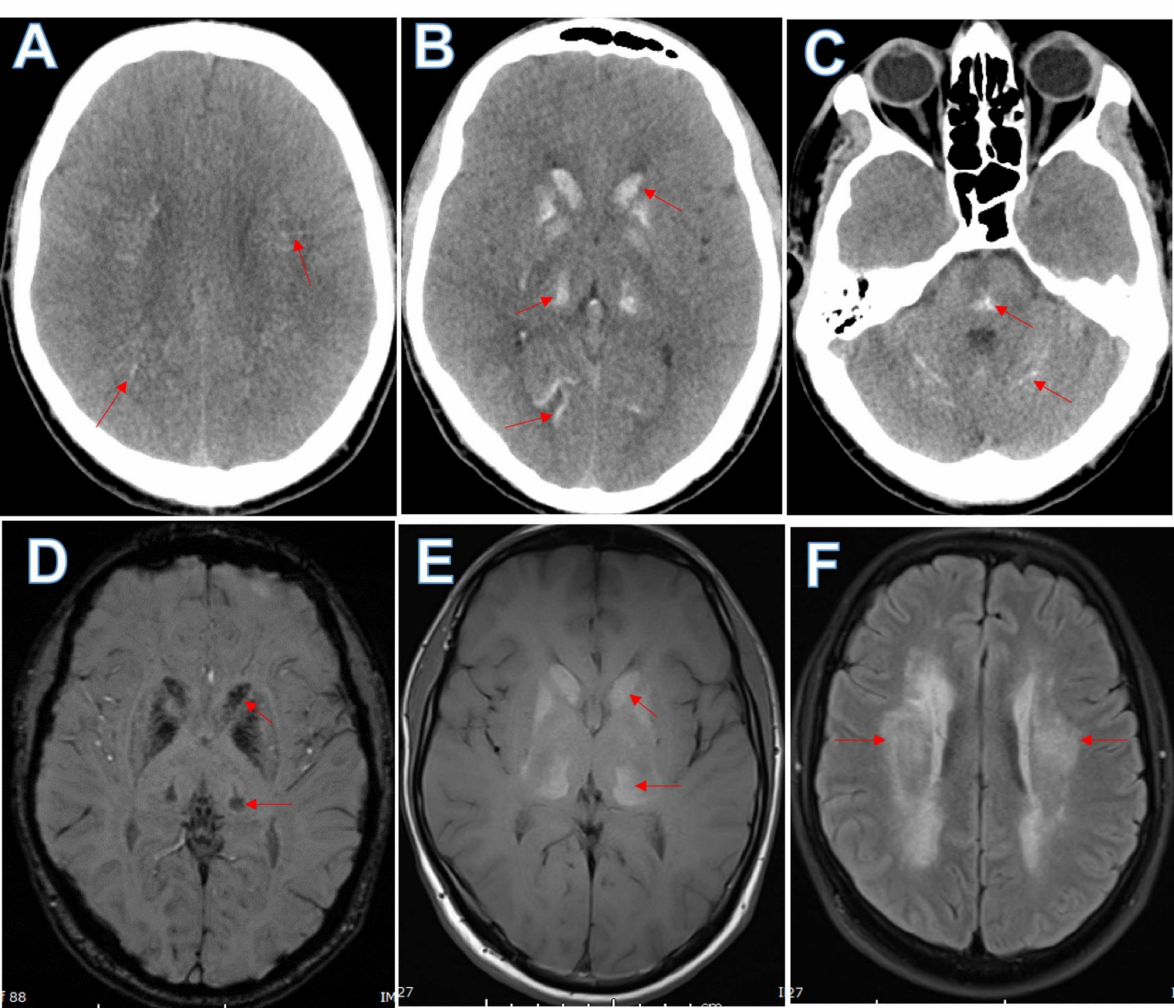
Brain imaging shows intracranial calcification Computed tomography (CT) scan of the brain shows, (A) - bilateral tiny deep white matter calcifications (arrows), (B) - prominent basal ganglia, thalami and posterior juxtacortical calcifications (arrows), and (C) - pontine, and cerebellar folia calcifications (arrows). Brain magnetic resonance imaging (MRI) demonstrates, (D) - expected blooming hypo intensity of the calcifications on susceptibility-weighted imaging (SWI), (E) - hyperintensity on T1 weighted imaging (arrows), and (F) - abnormal hyperintensity in the deep white matter on fluid-attenuated inversion recovery (FLAIR) sequence (arrows) of brain MRI, surrounding the tiny calcifications seen on the CT scan.
